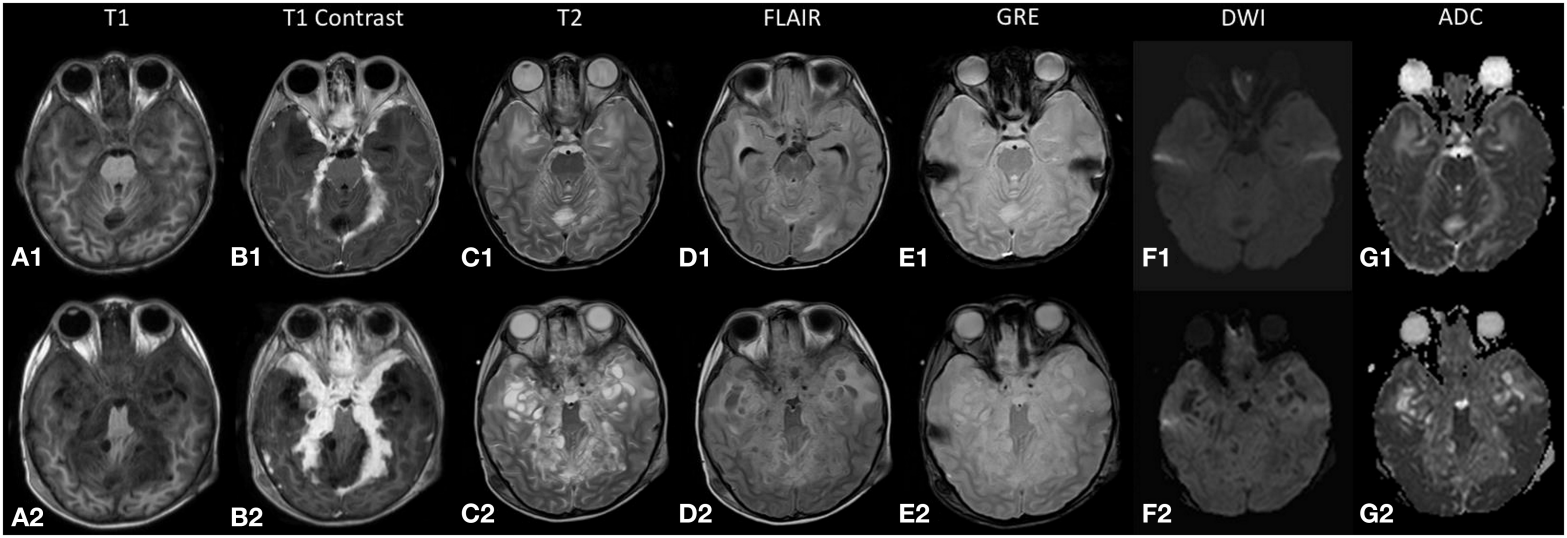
The top row (A1–G1) displays magnetic resonance (MR) images from the initial study during the first admission, and the bottom row (A2–G2) displays the corresponding images during the relapse 2 months later. The lesion (A1,A2) returns an isointense signal on T1-weighted images and (B1,B2) demonstrates enhancement on post-contrast images. (C2) High T2-signal cystic lesions are seen along the leptomeningeal surface that increased in size and number during the relapse. (D2) The internal signal of these cystic lesions is not entirely suppressed on fluid attenuation inversion recovery (FLAIR) sequence. (E1,E2) No blooming artifacts seen on gradient echo (GRE) sequence. (F1,G1;F2,G2) Restricted diffusion is not demonstrated in both studies.
